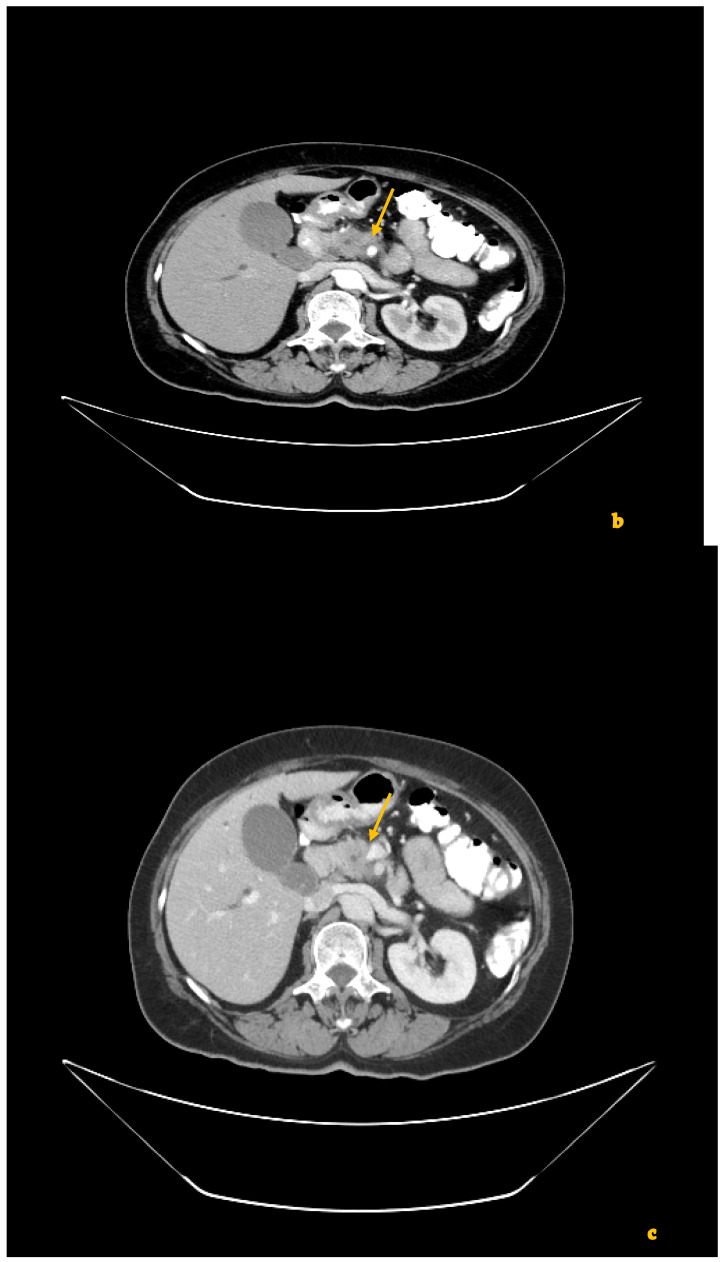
(a–c) Contrast-enhanced CT shows a locally advanced pancreatic cancer in the uncinate process (arrow), infiltrating the superior mesenteric vessels with mark deformation and retraction (a). After chemotherapy (b,c), there is still visible tissue close to the superior mesenteric vessels but to a lesser extent, specifically around the superior mesenteric artery (arrow) that seems no more retracted (b), while retraction persists in the superior mesenteric vein (arrow), probably due to fibrosis (c).
